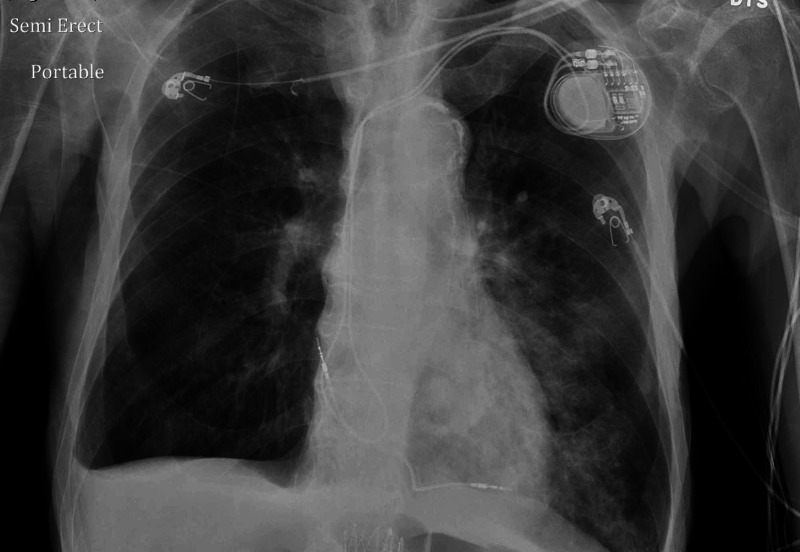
Chest X-ray showing left lower lobe infiltrates
